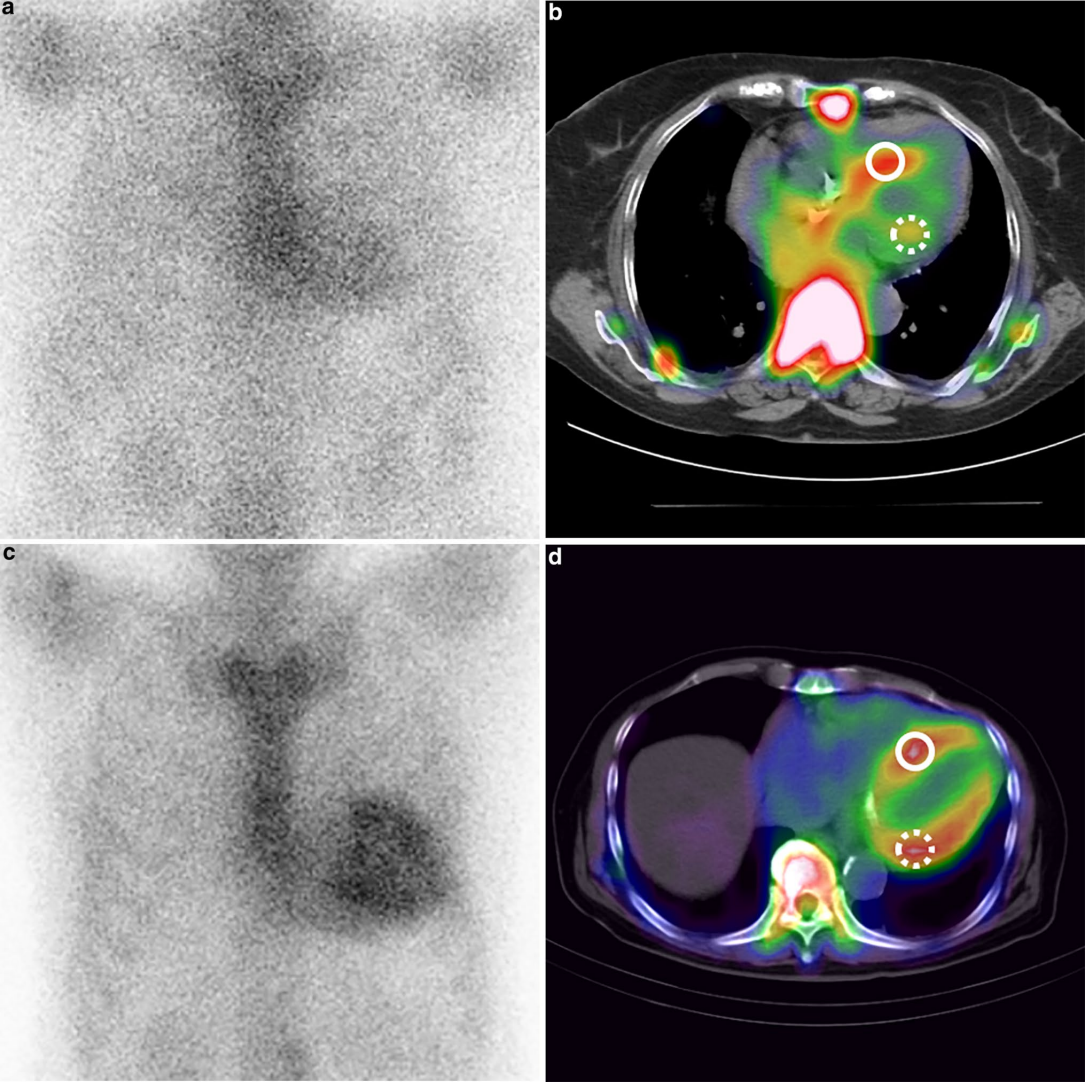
PYP planar and SPECT/CT images of two ATTRwt-CM patients. Circles indicate the region of interest for the highest PYP accumulation in the septal and lateral walls of the left ventricle. A, B An 86-year-old female patient with ATTRwt-CM had an hs-cTnT level of 0.027 ng/mL and an event-free survival of 1441 days. A The planar image shows mild PYP accumulation in the left ventricular myocardium with an H/CL of 1.41. B In SPECT/CT fusion image, PYP accumulation is obviously lower in the left ventricular lateral wall (dashed circle) with a La/C of 1.51 than that in the septal wall (circle) with a Se/C of 2.01. C, D A 79-year-old male patient with ATTRwt-CM had an hs-cTnT level of 0.0855 ng/mL and was hospitalized for heart failure on day 588 and died on day 1880. C The planar image shows diffuse PYP high accumulation in the left ventricular myocardium with an H/CL of 1.99. D On SPECT/CT fusion image, the PYP accumulation is slightly higher in the lateral wall (dashed circle) with a La/C of 2.80 than the septal wall (circle) with a Se/C of 2.52
